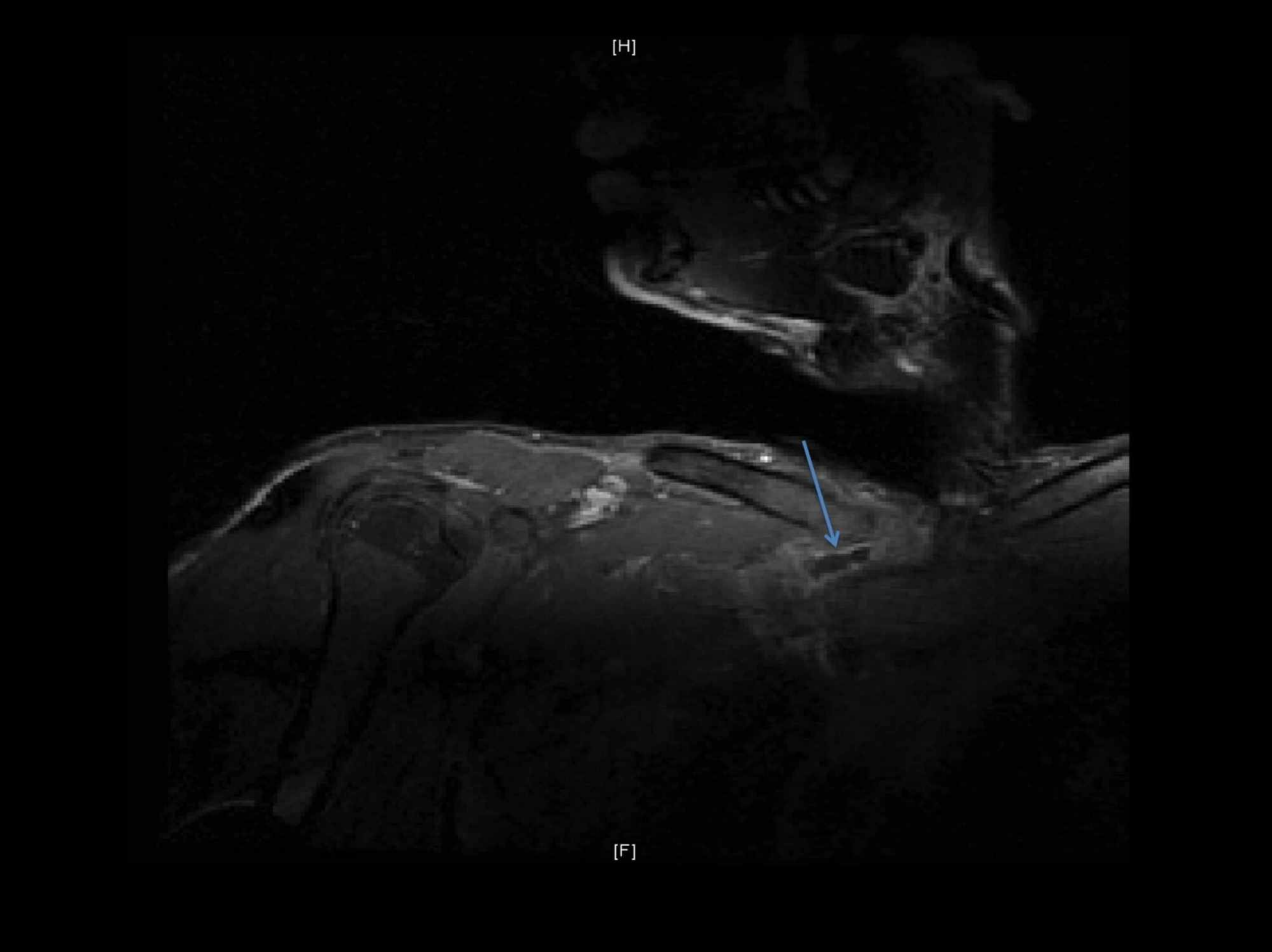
MRI of the right SCJ with contrast. Follow-up MRI of the right SCJ with contrast demonstrated old postsurgical changes in the SCJ and surrounding tissues but no significant contrast enhancement is visualized which endorsed the resolution of the infectious process. SCJ, sternoclavicular joint
